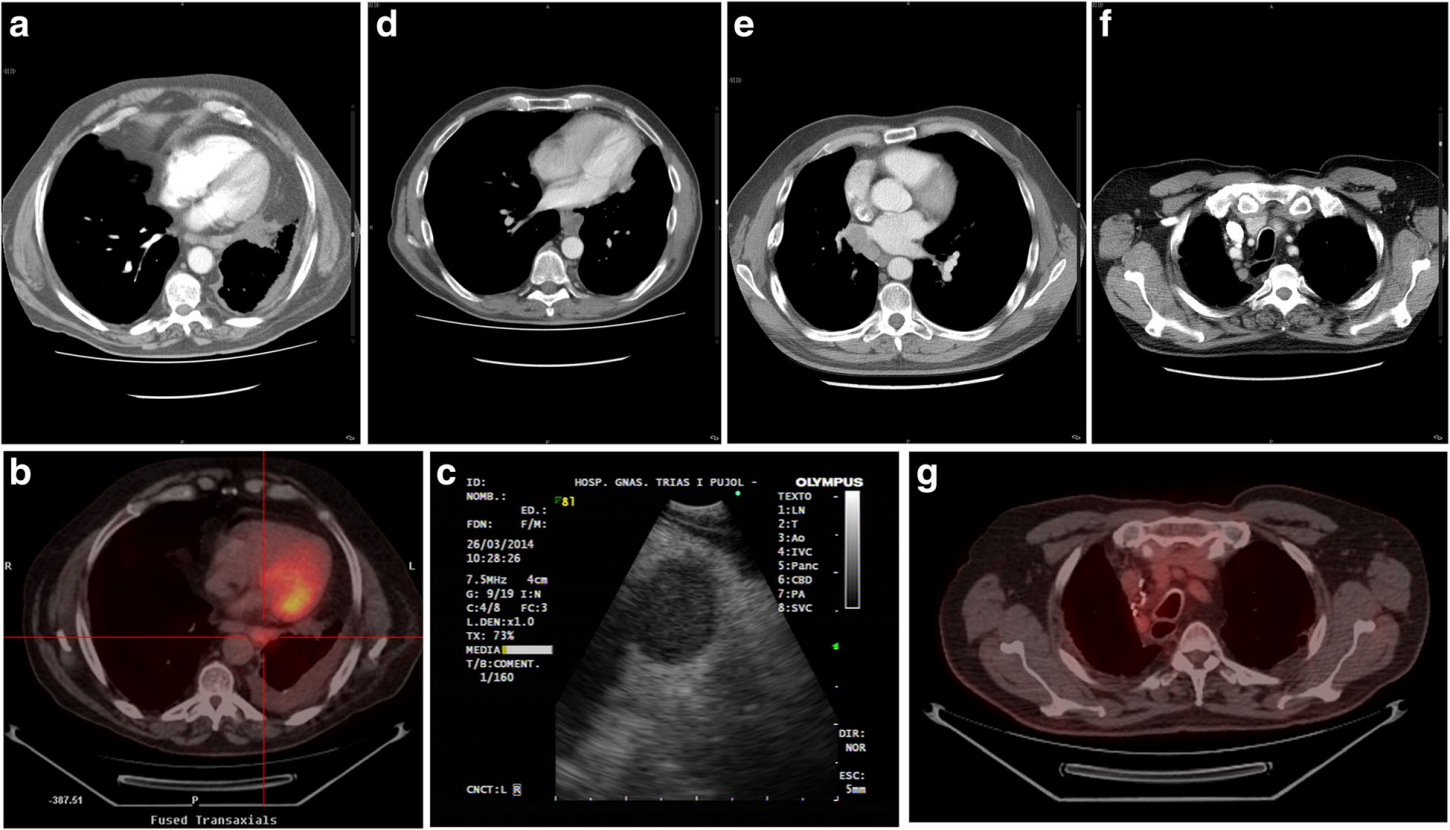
Four cases of recurrence diagnosed by EUS-B-FNA: *Case 1* (a, b, c): a 68-years old man with a stage IIA squamous-cell carcinoma in LLL treated by means of lobectomy presented a pleural effusion on the first CT control 6 months after the surgery, the PET/CT showed a high uptake on a 8 left station node, an EUS-B-FNA confirmed recurrence. *Case 2* (d): a 55-years old man with a previous stage IA NSCLC-NOS in RUL treated by means of wedge resection presented an 8 left lymphadenopathy on the first CT control 6 months after the surgery. *Case 3* (e): a 40-years old man with a carcinoid on middle lobe treated by means of RLL and ML bilobectomy presented a mass/nodal enlargement on right 8 station that invaded the pulmonary veins, the diagnosis was consistent with atypical carcinoid. *Case 4* (f, g): a 71-year old man with a stage IIA adenocarcinoma in RUL treated by means of lobectomy presented a 2R adenopathy in a CT 21 months before the surgery. Although being a paratracheal node the approach was easier through the esophagus
